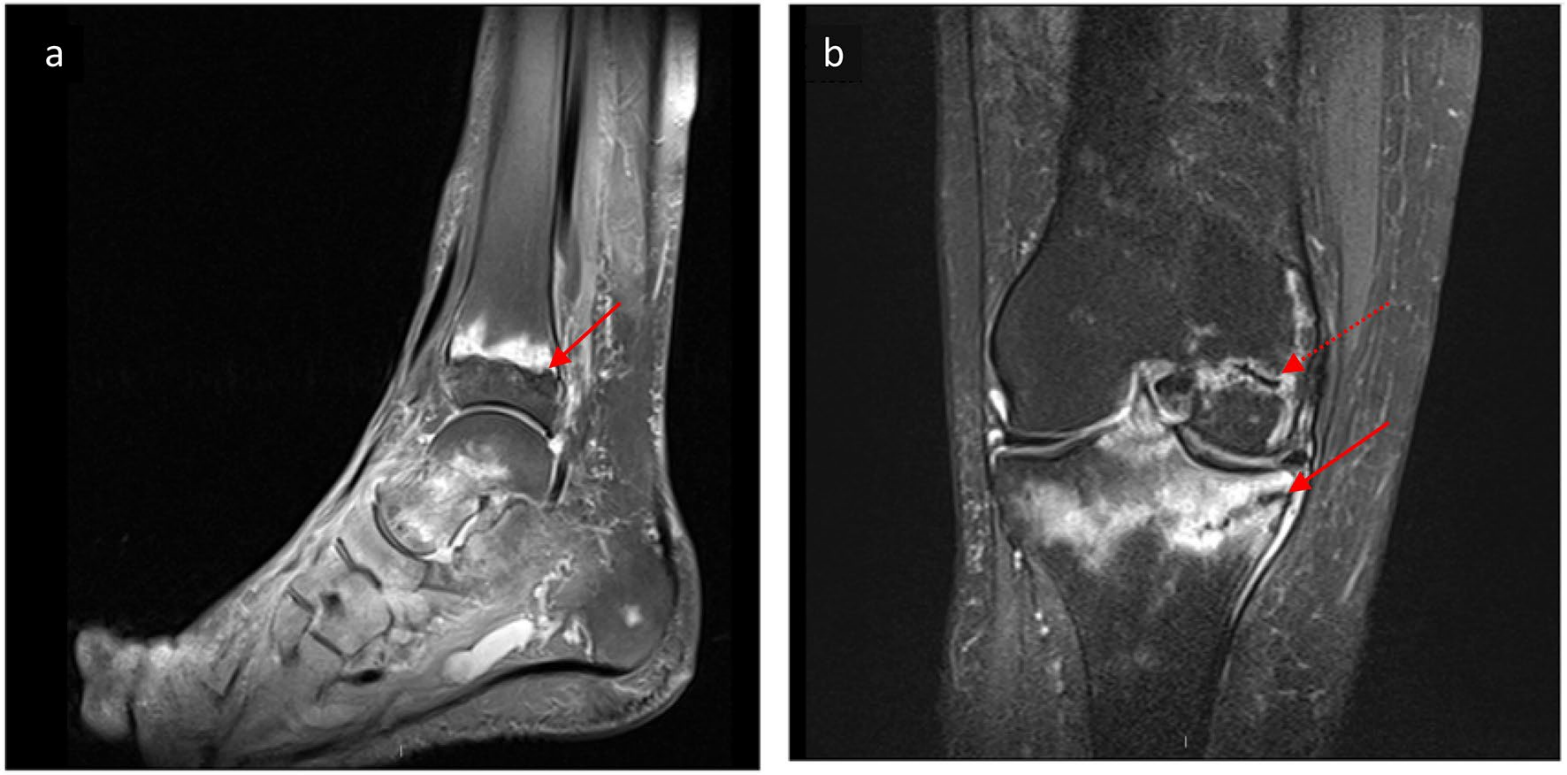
Radiographic features of Methotrexate osteopathy. MRI images of rheumatoid arthritis patients on long-term methotrexate patients who developed insufficiency fractures with band-like fracture lines along the growth plate a red arrow points to distal tibia metaphysis insufficiency fracture b upper (dotted) red arrow points to distal femur metaphysis fracture and lower (continuous line) red arrow points to proximal tibia metaphysis fracture (Color figure online)
